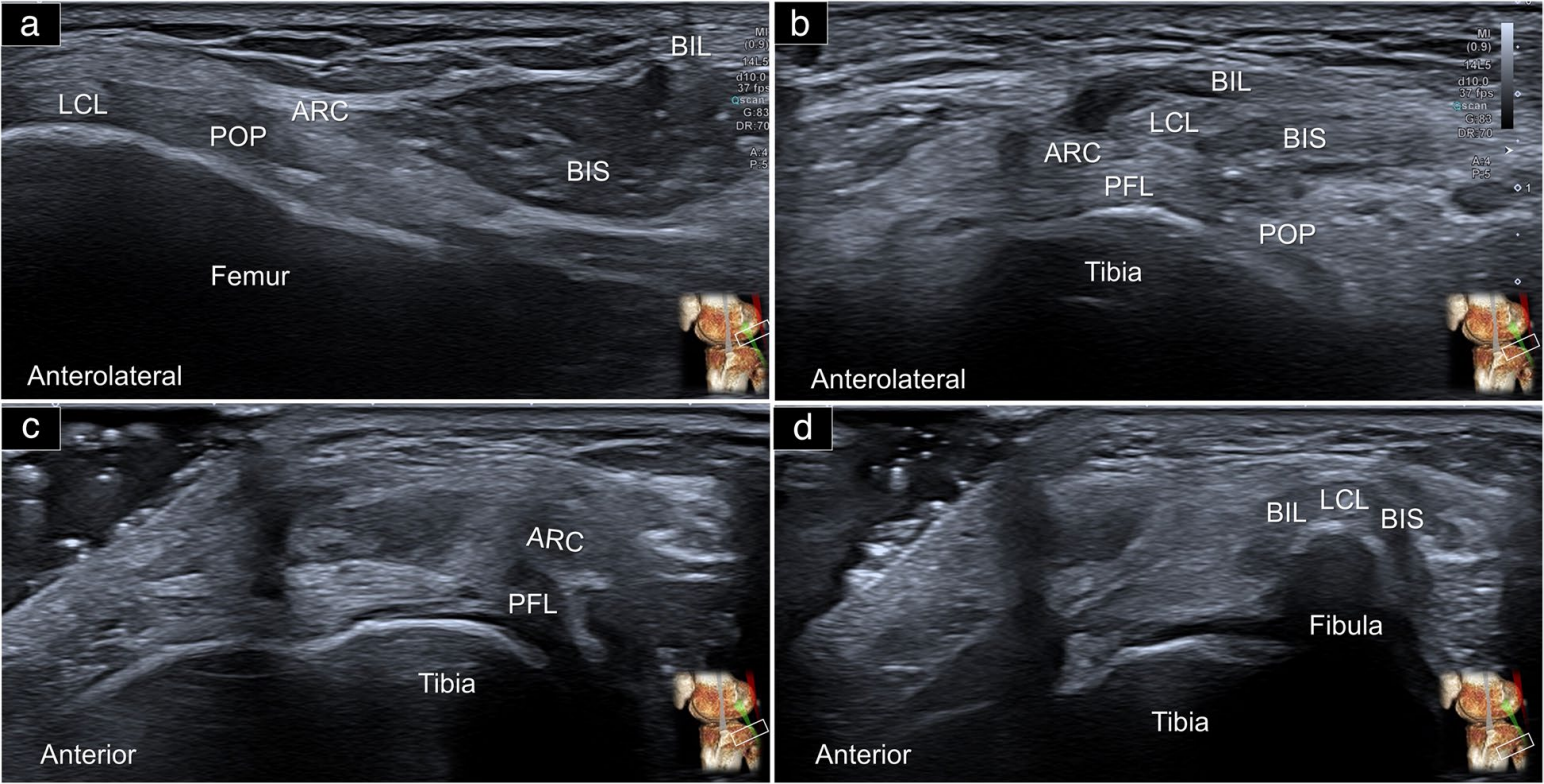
Sonographic imaging displays the posterior lateral corner of the knee in the transverse plane, at the level of the lateral femoral condyle (a), across the tibial plateau (b), over the proximal tibia shaft (c), and at the fibular head (d). This comprehensive view facilitates the visualization of both the arcuate and popliteofibular ligaments. LCL: lateral collateral ligament; BIL: long head tendon of the biceps femoris; BIS: short head tendon of the biceps femoris; ARC: arcuate ligament; PFL: popliteofibular ligament
